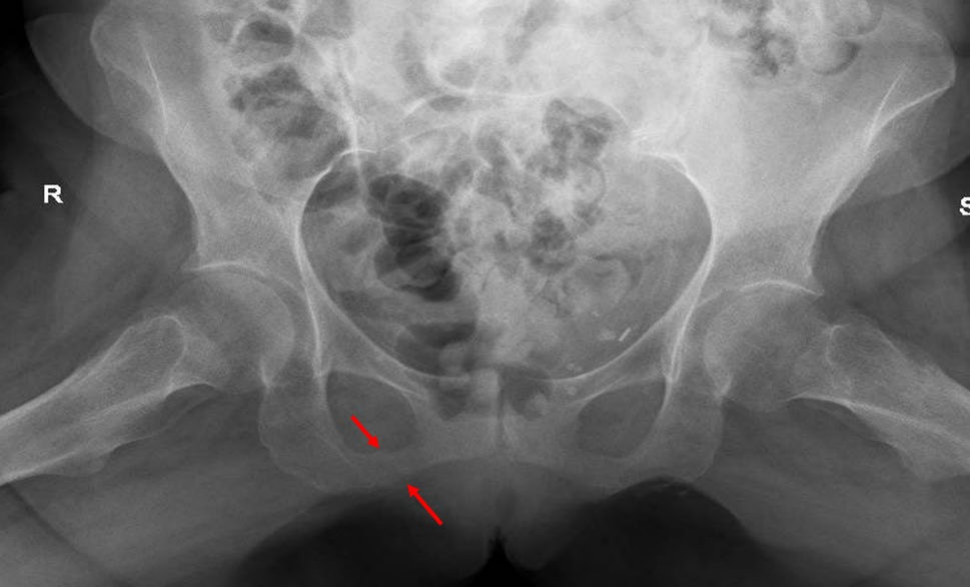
X-rays of the shoulders and right knee
